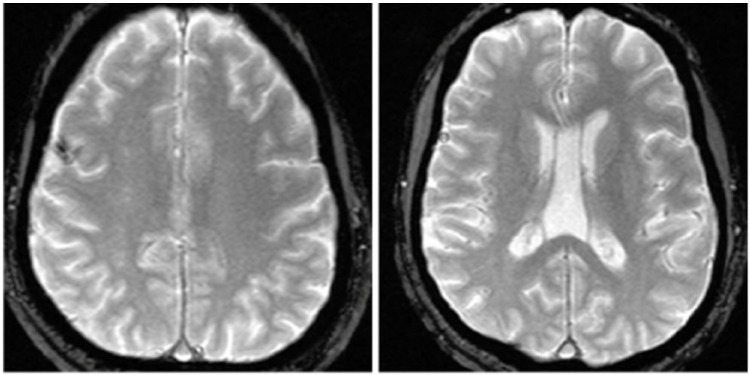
Axial section T2 Flare MRI image showing infarction of the right posterior
